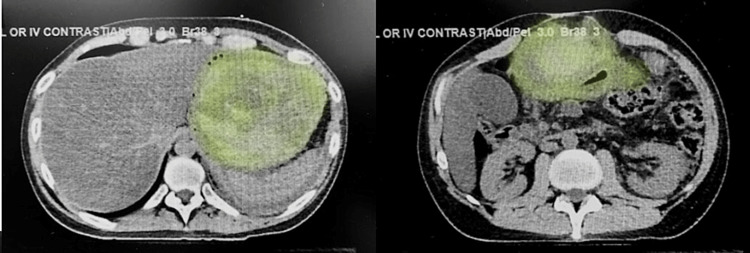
Contrast-enhanced CT scan of the abdomen: axial view The images show a large pancreatic pseudocyst (green overlay) occupying almost the entire left half of the abdomen and protruding anteriorly CT: computed tomography
